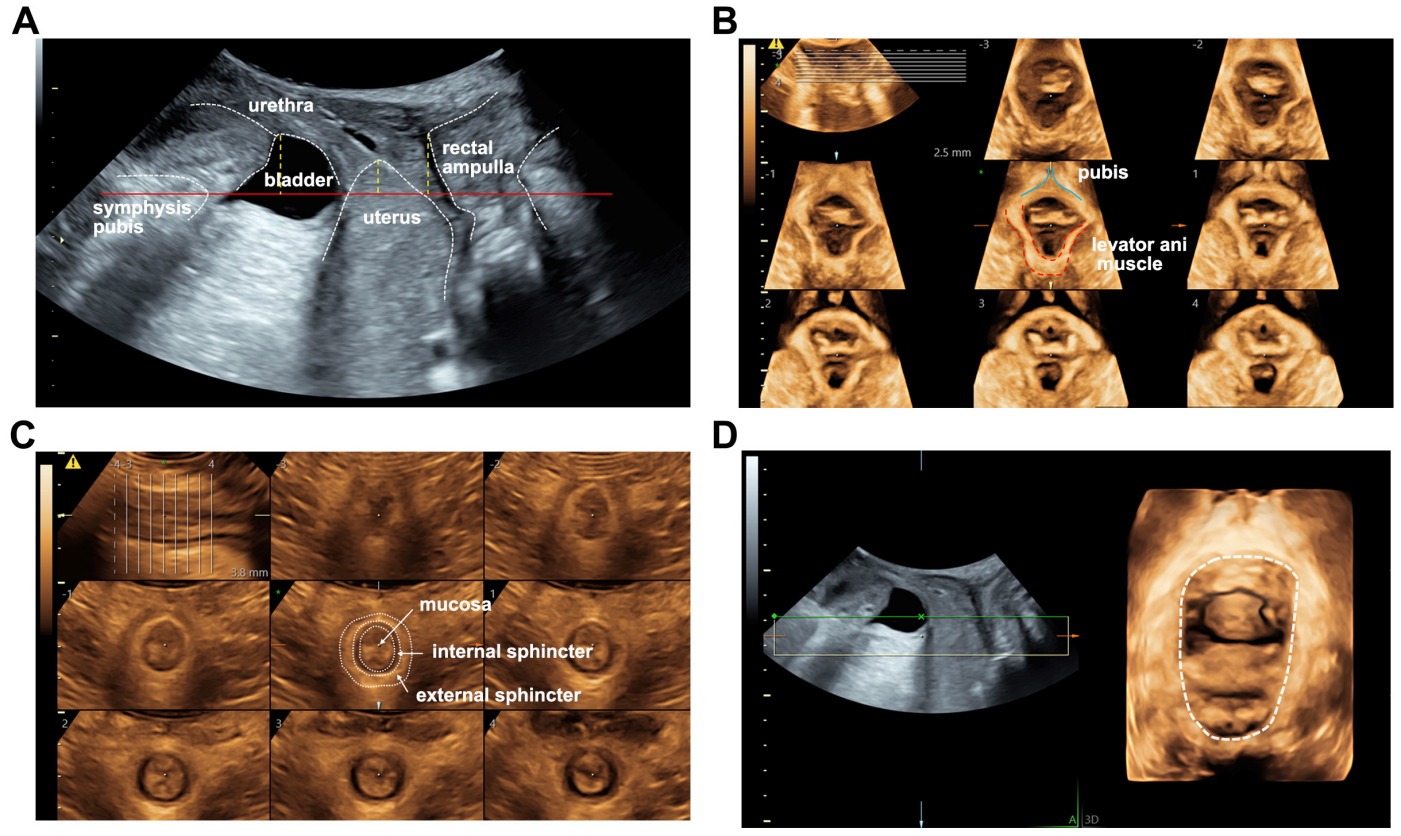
Pelvic floor ultrasound images of a 47-year-old woman with constipation. (A) Mid-sagittal gray-scale image indicated the vesicocele and uterine prolapse. The horizontal line (the red solid line) was the reference line passing through the posterior inferior margin of the symphysis pubis. The vertical line (the yellow dotted line) indicated the distance from the bladder, uterus, and rectal ampulla to the reference line. (B) Tomography ultrasound images showed the left side levator ani muscle was trauma. (C) Tomography ultrasound images of the anal sphincter was normal. (D) The levator hiatus area (the white line) at the maximal Valsalva maneuver was over 20 cm^2^.
